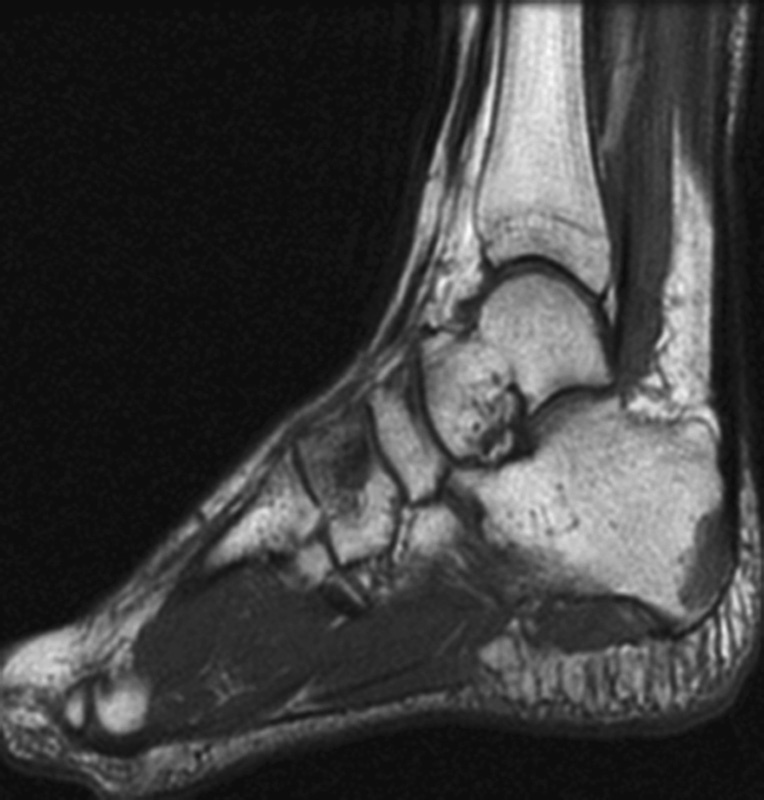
Hypointense lesions in calcaneus and cuneiform (T1-weighted magnetic resonance imaging section).
